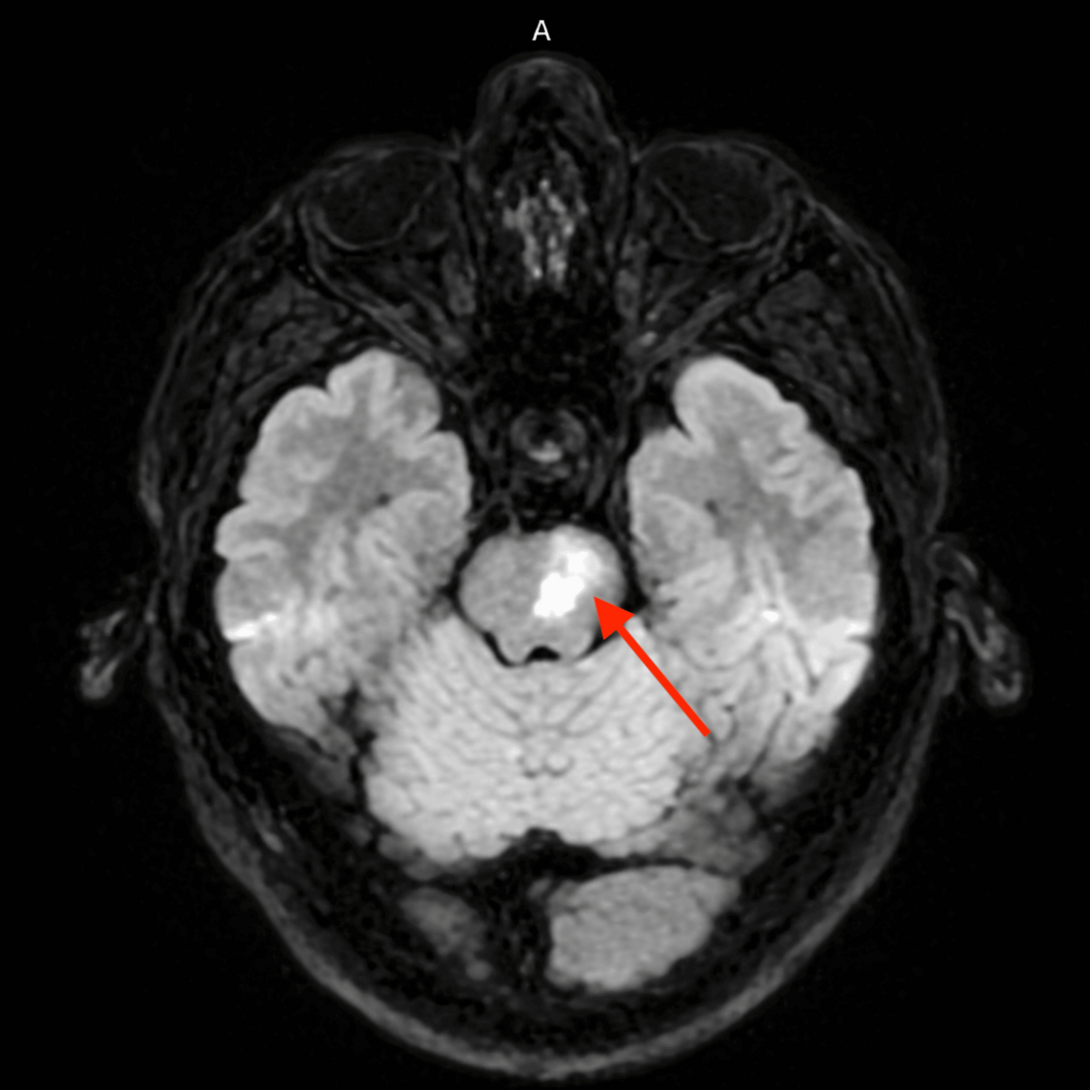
Brain MRI demonstrating a subacute infarct involving the left pons (arrow), corresponding to the patient's right-sided weakness, facial droop, and ataxia.
